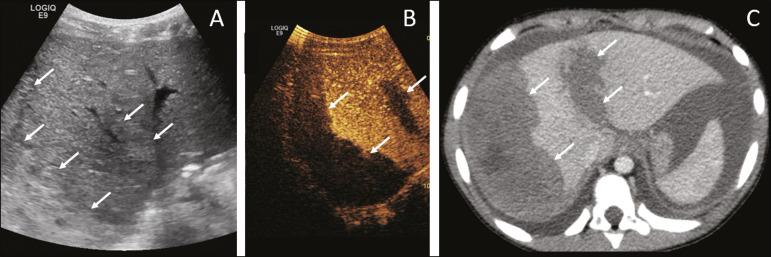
A nine-year-old patient with abdominal trauma from a domestic accident. A: Conventional ultrasound showing slightly hypoechoic and heterogeneous areas in the right lobe of the liver, with ill-defined borders (arrows). B: After administration of contrast medium, ultrasound clearly showed a laceration in the right lobe (arrows). C: Computed tomography scan acquired on the same day, showing lacerations (arrows).
